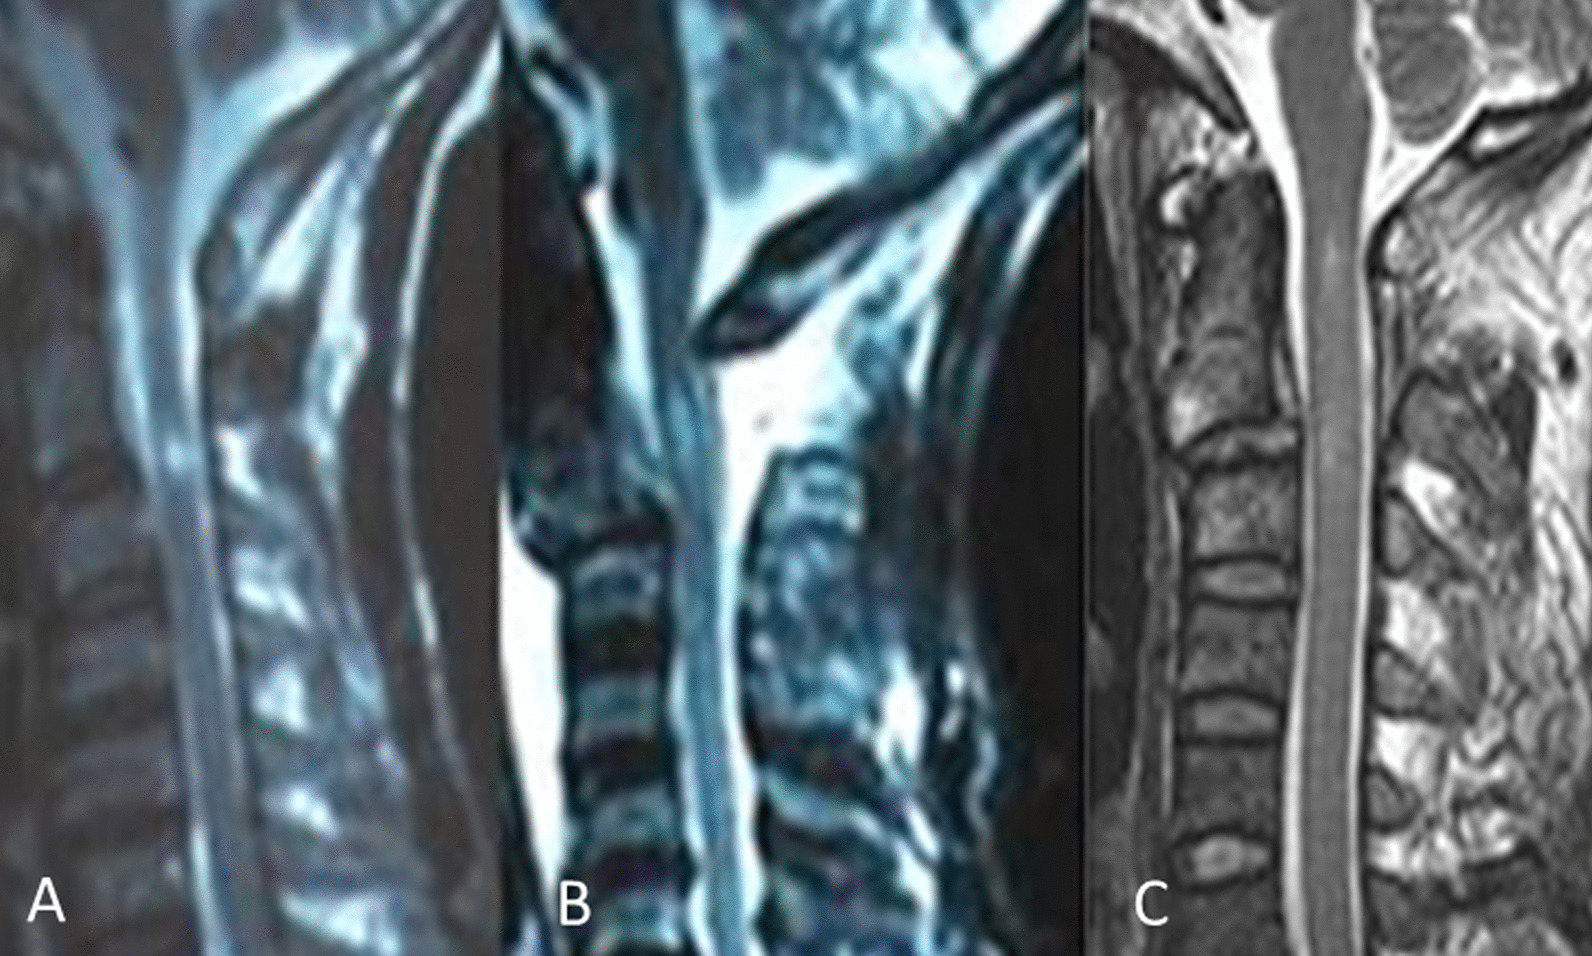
Magnetic resonance images showing the location and range of signal changes on T2-weighted images in the spinal cord. One high signal at the level of C2–C3 disc (A). One high signal at the C2 level (B). High signals at the C2 and C2–C3 disc levels (C)
